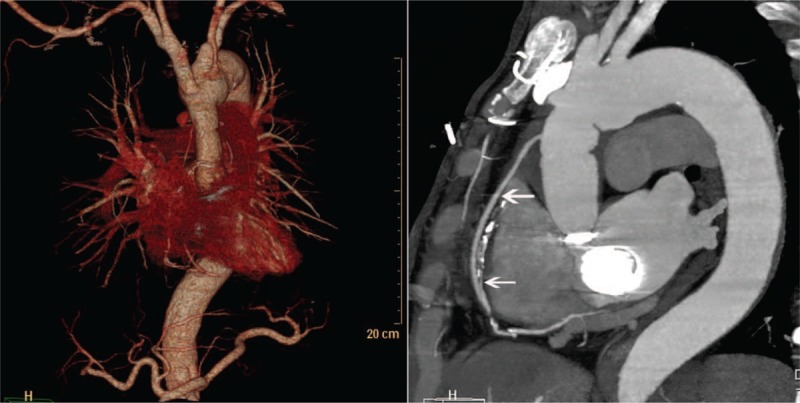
Computed tomography angiography showing that the ascending aorta is normal and that the saphenous vein graft to the right posterior descending artery remains patent (arrows).
